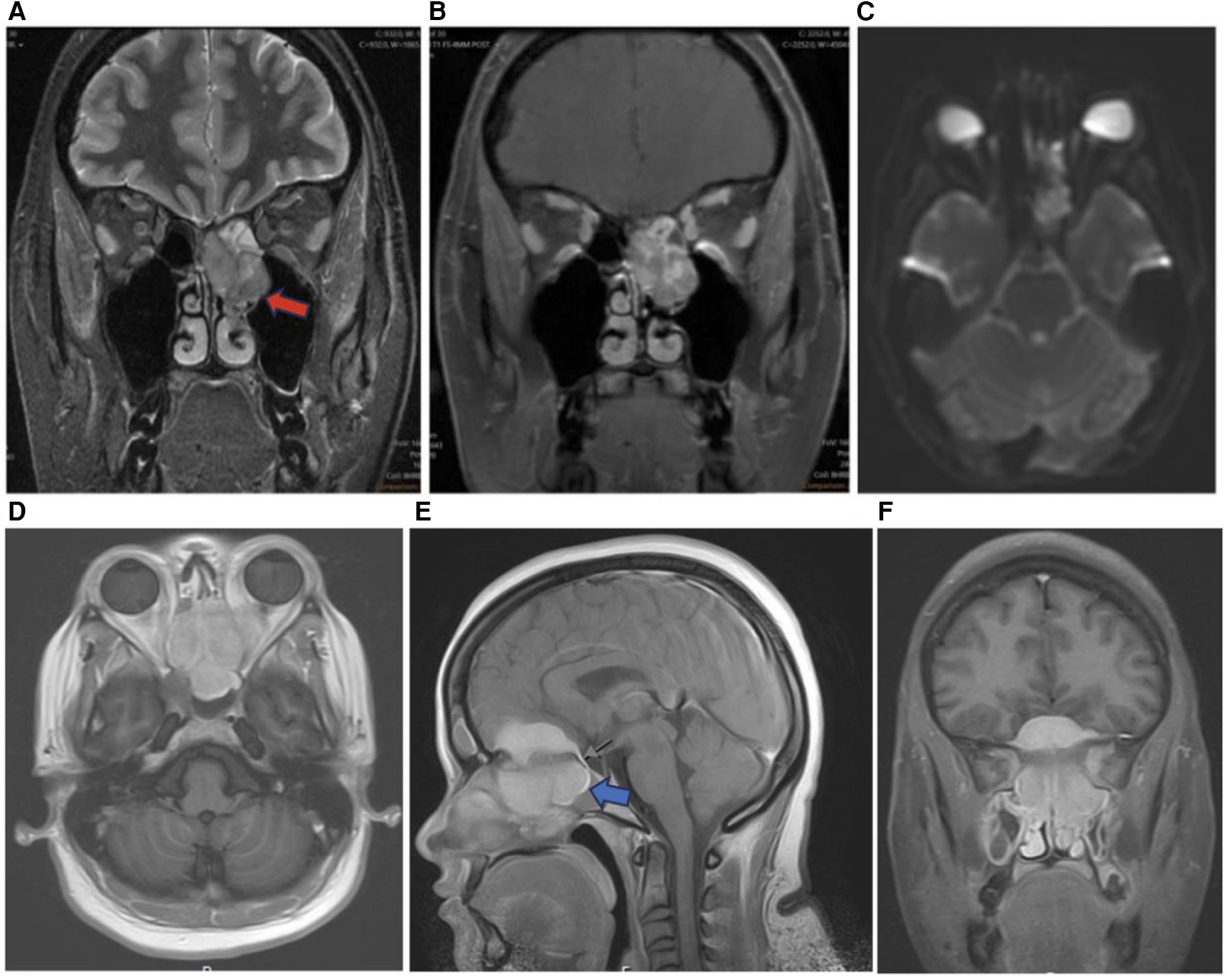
Sinonasal soft tissue tumors. Coronal T2 (A), coronal T1 + C (B), and axial DWI (C) showing sinonasal rhabdomyosarcoma centered in the left ethmoid (red arrow) demonstrating enhancement and diffusion restriction. Axial T1 + C (D), sagittal T1 + C (E), and coronal T1 + C (F) MR images showing a homogenously enhancing meningioma centered in the midline of the anterior skull base with extracranial components in the ethmoid sinuses (blue arrow), nasal cavities, sphenoid sinuses, and medial extraconal space of the orbits.
